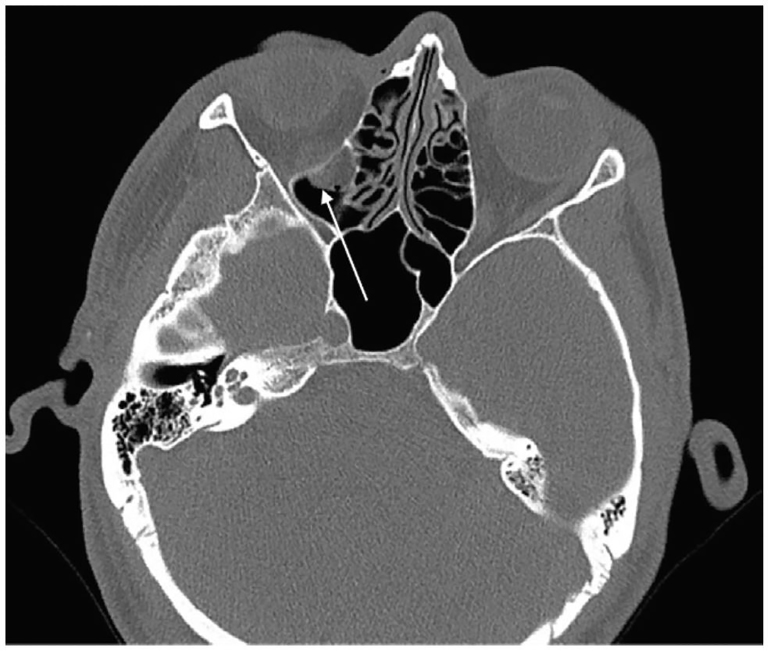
Axial non-enhanced CT in bone window: Soft tissue density again noted in the maxillary antrum (arrow). No definite fracture.
